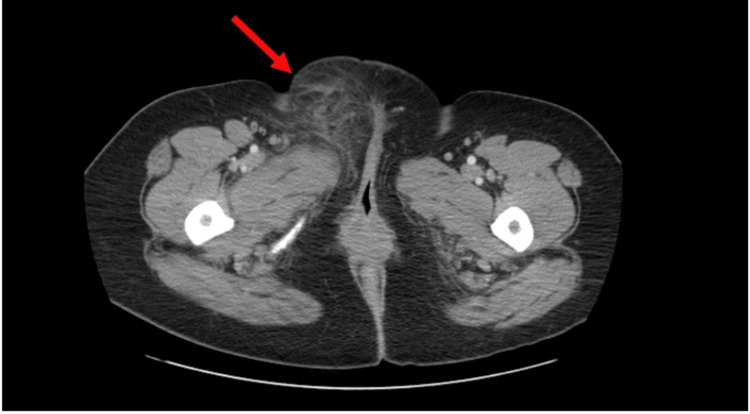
Computerized tomography (CT) of the abdomen and pelvis reveals extensive edema in the right groin and right vulva with a 1.2 cm early abscess.
